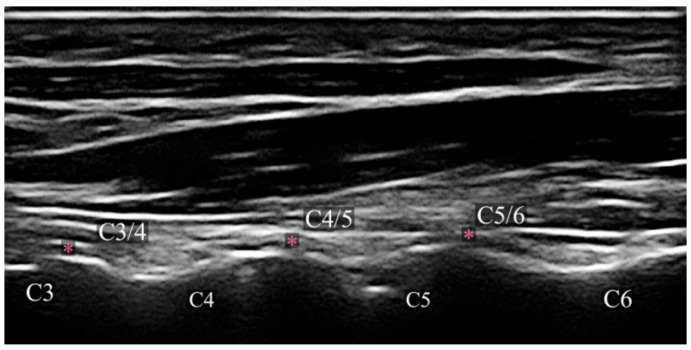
Sagittal ultrasound view of the cervical spine demonstrating the articular pillars. The articular pillars of C3, C4, C5, and C6 vertebrae are visualized as a continuous wavy hyperechoic line. The cervical medial branch target points at the waist of each articular pillar are marked with asterisks (*) at the C3/4, C4/5, and C5/6 levels.
